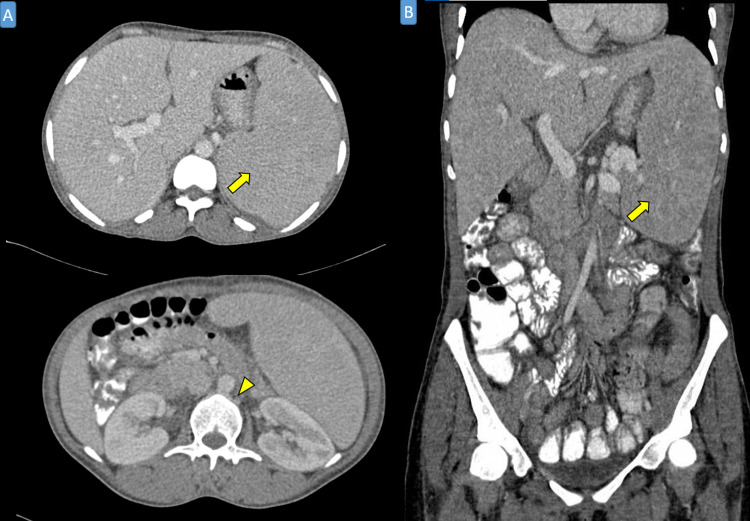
Abdominal computed tomography CT scan with contrast in the axial (A) and coronal (B) with venous phase that shows severe splenomegaly (yellow arrow) with no evidence of periaortic lymph nodes enlargement (yellow arrowhead).
